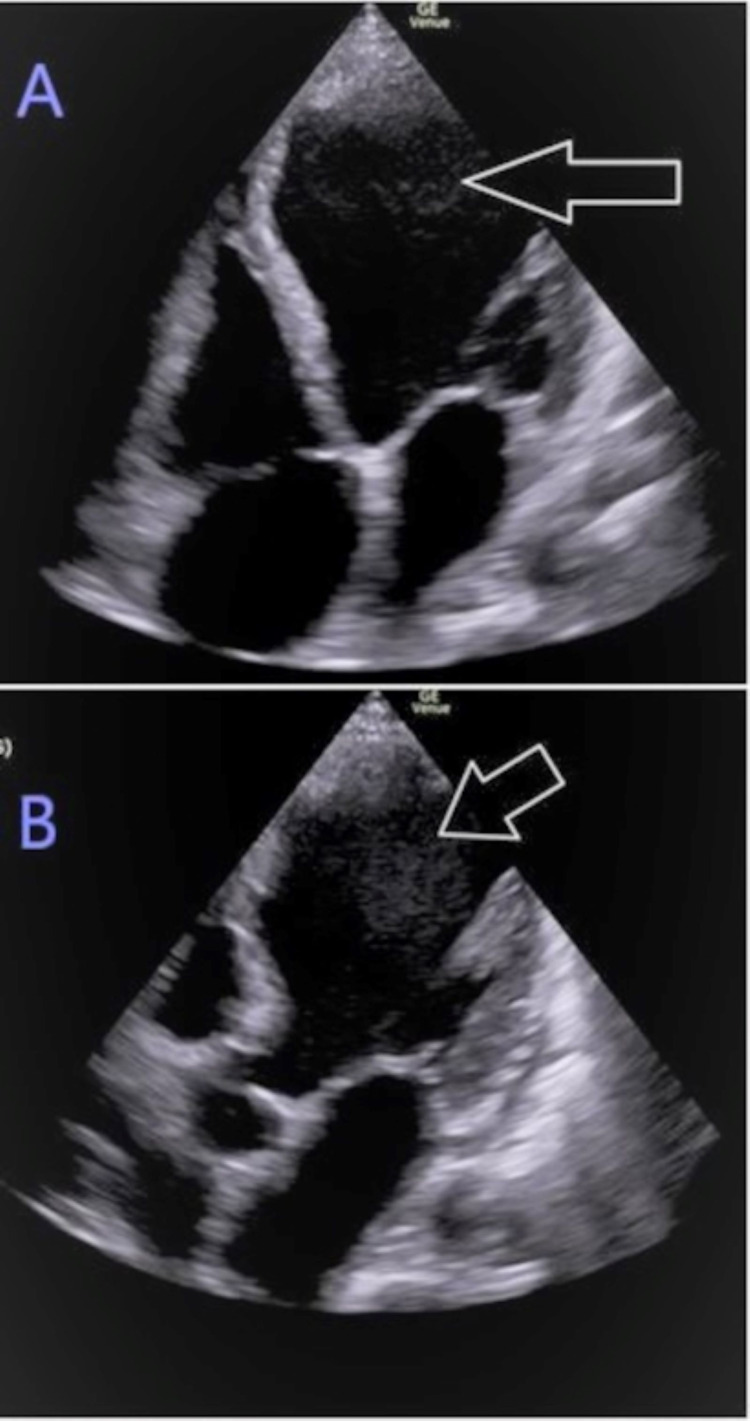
POCUS ECHO showing dilated cardiomyopathy and intraventricular thrombus (arrow). (A) apical four-chamber. (B) Apical five-chamber views. POCUS: point-of-care; ECHO: echocardiography
